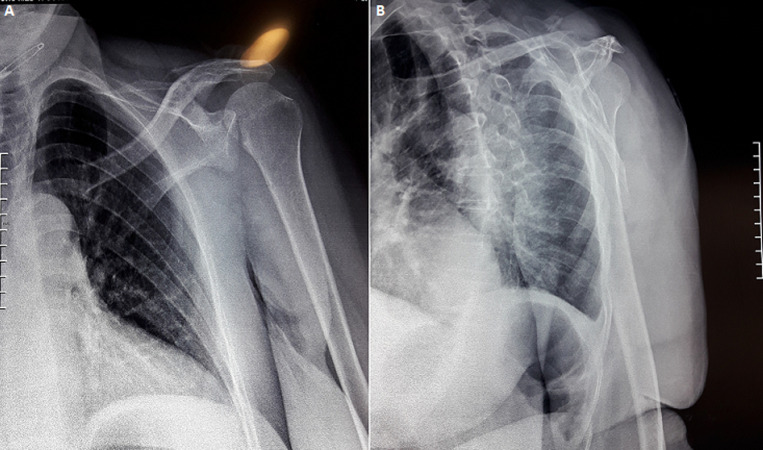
radiographs of the left shoulder: A) frontal radiograph of shoulder showing double contour of humeral head “trough sign” due to a reverse Hill-Sachs lesion; B) lateral view showing the humeral head projected behind the glenoid
